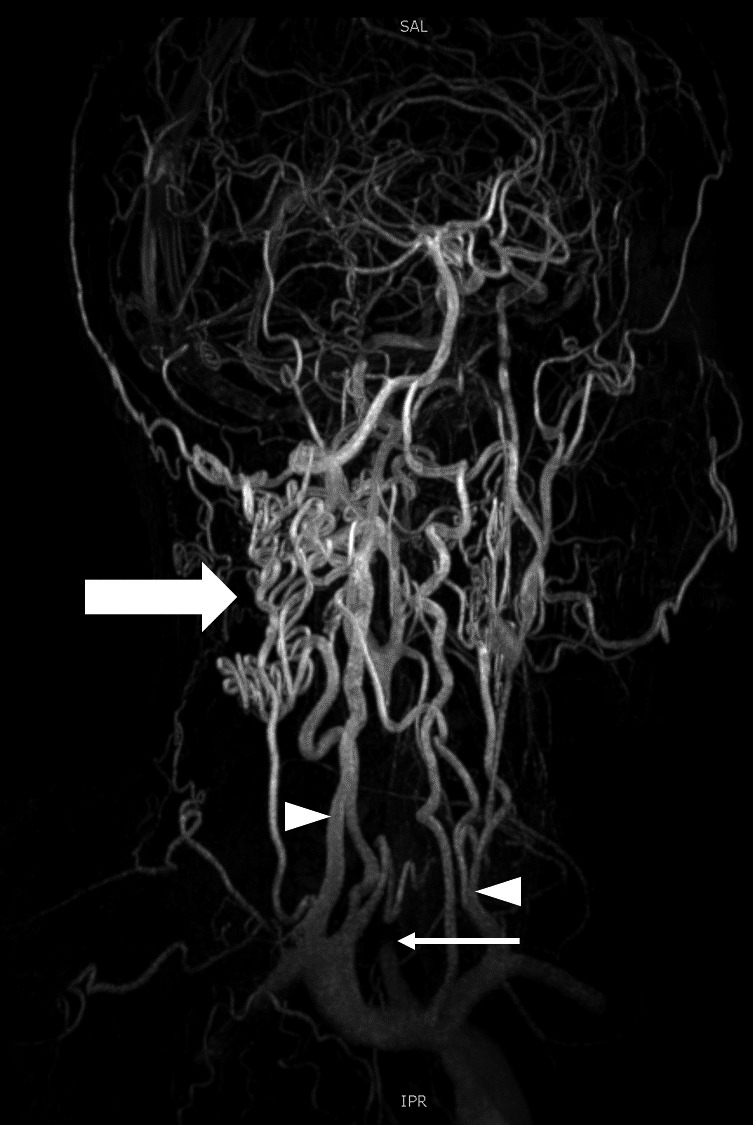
Contrast‐enhanced MRI angiography, maximum intensity projection (MIP) images of the neck arterial system. The vertebral arteries are prominent (white arrowheads) with numerous collaterals in the occipital region and prominence of the posterior circulation (white block arrow). Occlusion of the right common carotid artery is seen (white arrow)
